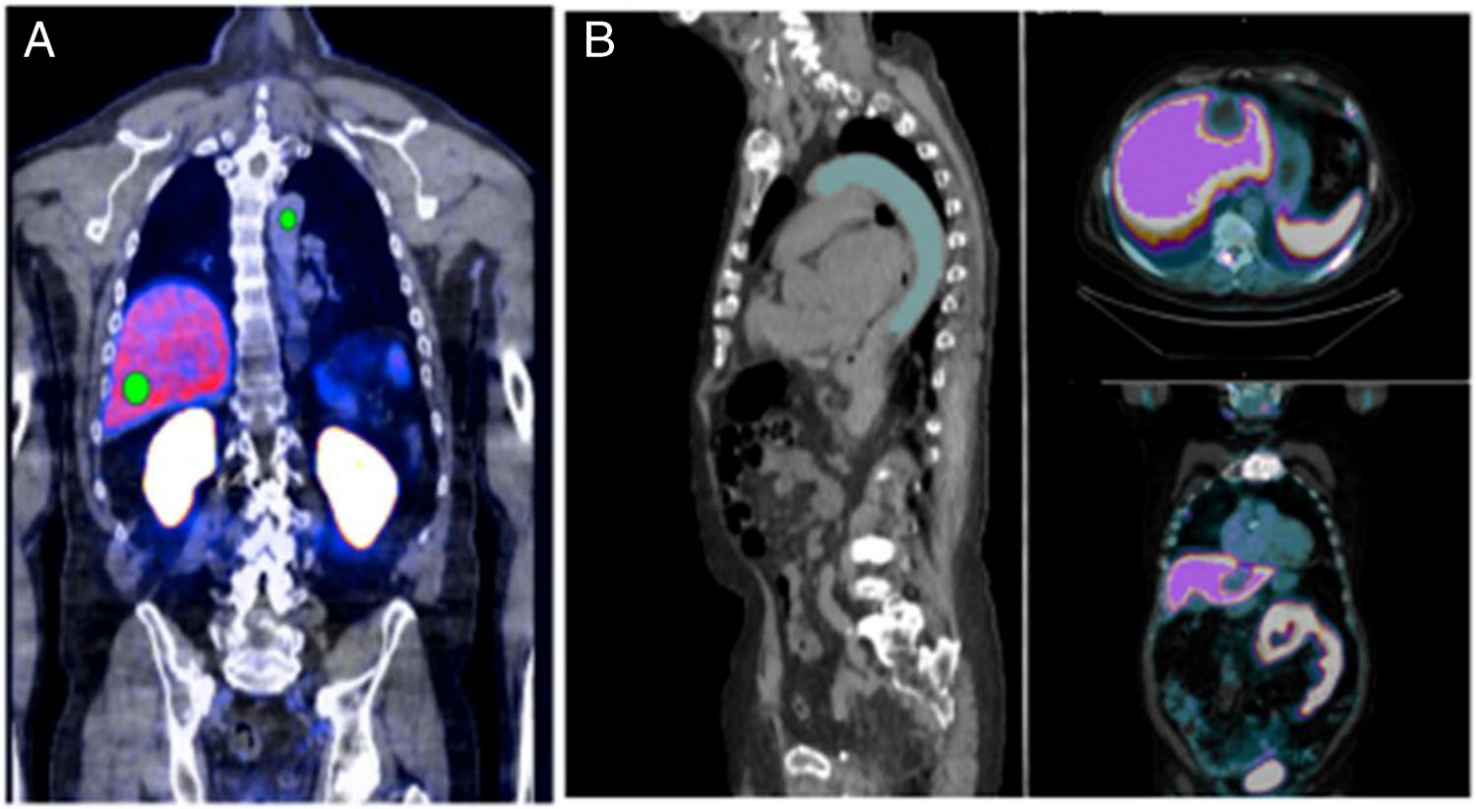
Manual placement of fixed ROI on a selected slice and location of liver and aorta to obtain SUVmean (A), against the automated mean reference organ uptake of liver and blood pool (aorta) (B) facilitated by the volumetric automated segmentation of the reference organs
